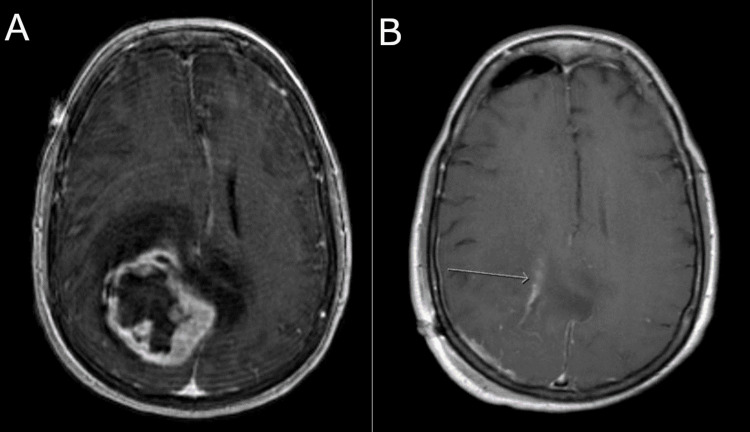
MRI of the brain. (A) Preoperative and (B) postoperative T1 MRI (axial view) with contrast. Postoperative imaging shows near gross total resection. MRI: magnetic resonance imaging
